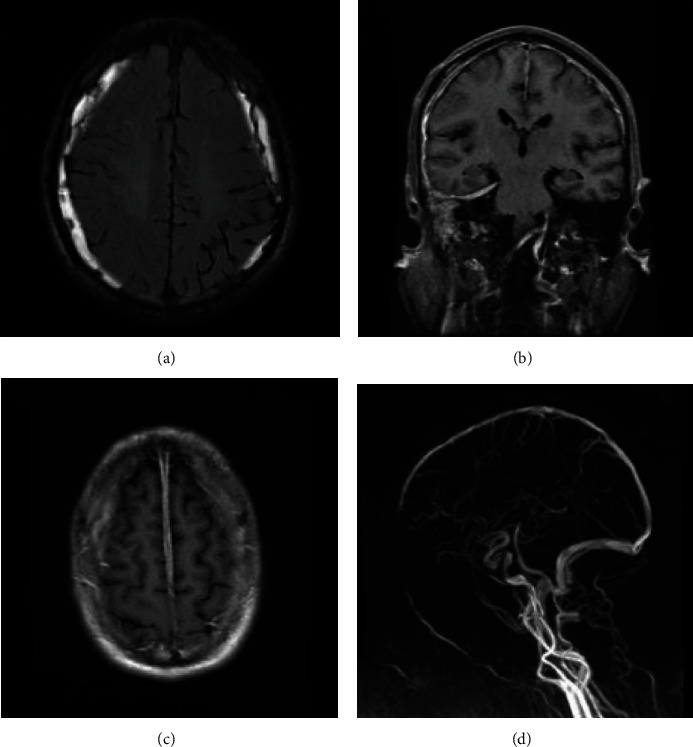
Hypertrophic pachymeningitis with subdural hemorrhage (Case 9). Bilateral subdural hemorrhage appears on axial T2-weighted fluid-attenuated inversion recovery imaging (a). Coronal T1-contrasted MRI reveals diffusion enhancement of the dura and right mastoiditis (b) and axial T1-contrasted MRI shows enhancement of the peripheral margin of the dura without central enhancement in frontal dura mater (c). Right otomastoiditis appears on MRI (b) and magnetic resonance venography was unremarkable (d).
